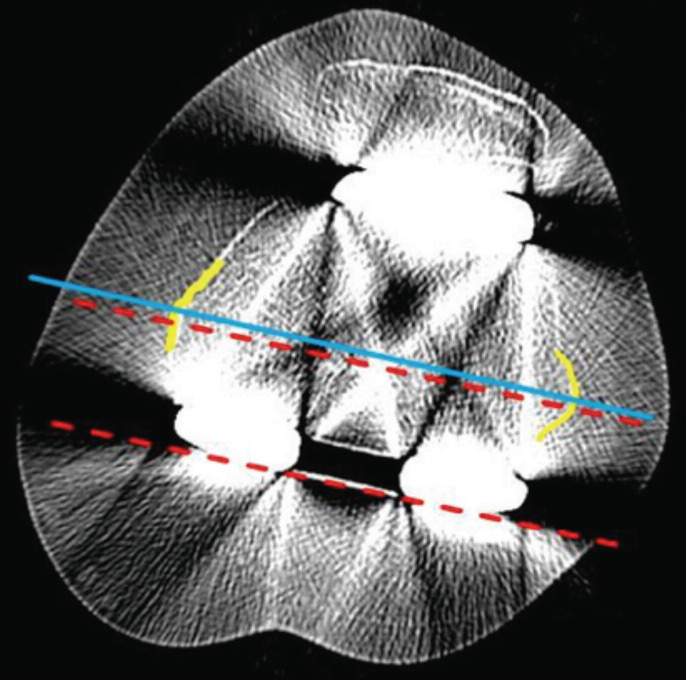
Computed tomographic image showing the measurement of the post-operative femoral component rotation (CT-FRA). The CT-FRA is the angle between the transepicondylar axis (blue line) and the posterior condylar axis of the femoral component (red dashed line).
